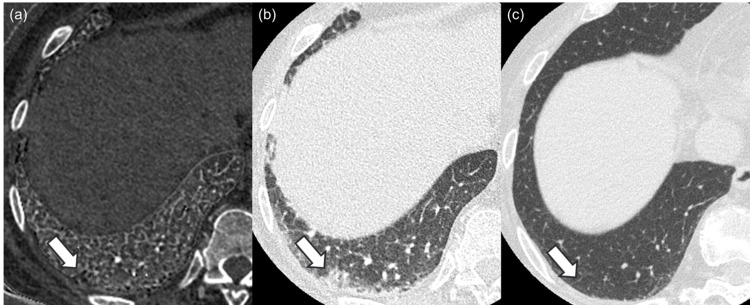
Images of a patient diagnosed with systemic sclerosis. The iodine map (a) and monochromatic image (b) of a 61-year-old female patient diagnosed with systemic sclerosis. Bronchiectasis and airspace enlargement, which are presented as black tiny structures in the region surrounded by white arrows, are more prominent in the iodine map compared to the monochromatic image, and readers 1, 2, and 3 rated 1 (iodine map is prominent), 1, and 2 (iodine map is strongly prominent) in side-by-side qualitative evaluation, respectively. Bronchiectasis and airspace enlargement were ameliorated in the evaluated region in the follow-up CT examination (c), which was performed after 490 days. Window level/width for iodine map (a) and CT images (b, c) were 15/110 mgI/ml and –600/1600 Hounsfield unit, respectively.
